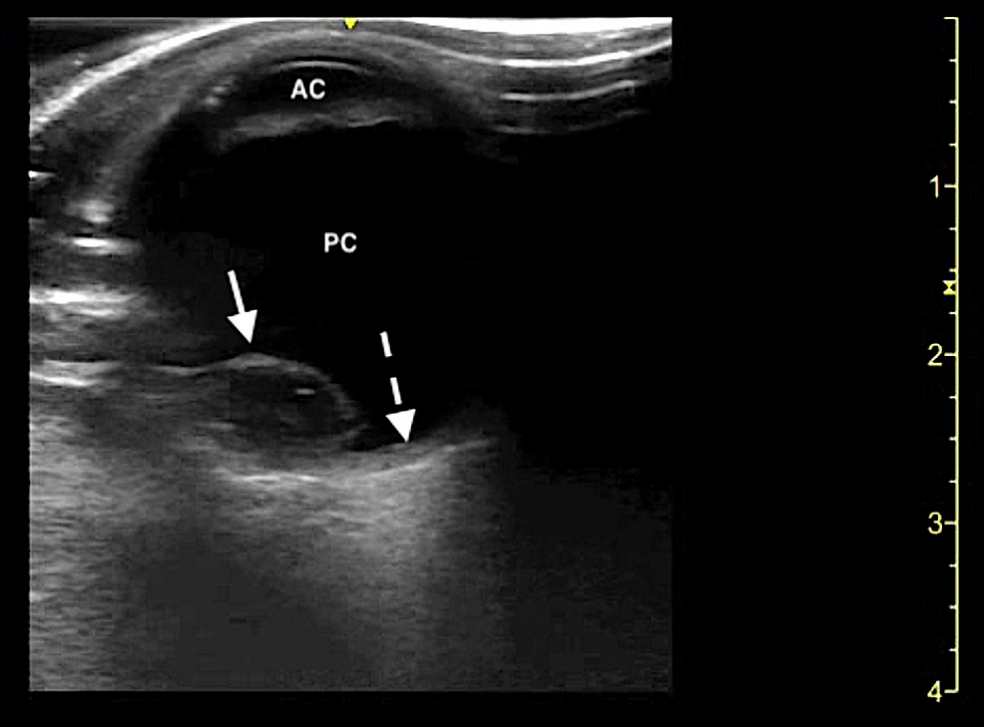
Ocular point-of-care ultrasound demonstrating a posteriorly dislocated lens. AC: Anterior chamber; PC: Posterior chamber; Dislocated lens (solid arrow); Retina (dotted arrow).
